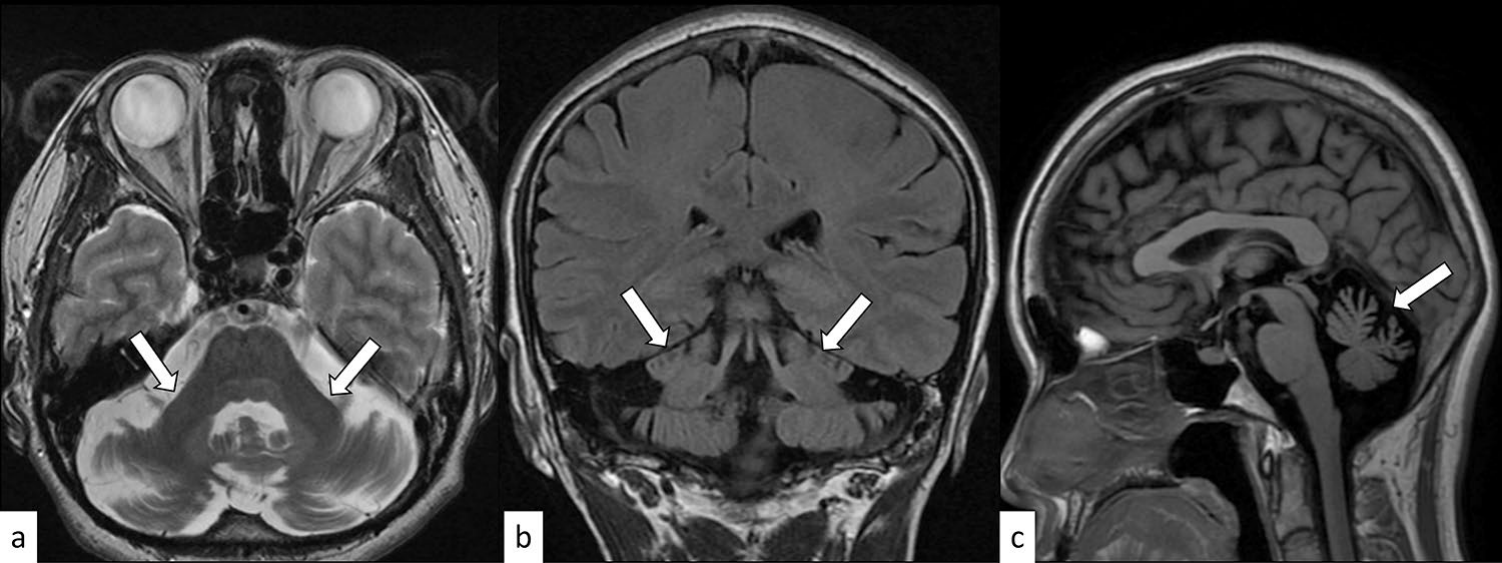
A 50-year-old man with SCA8. T2-weighted axial image a, FLAIR coronal image b, and T1-weighted sagittal image c show cerebellar atrophy without atrophy of the brainstem and cerebrum (arrows)
